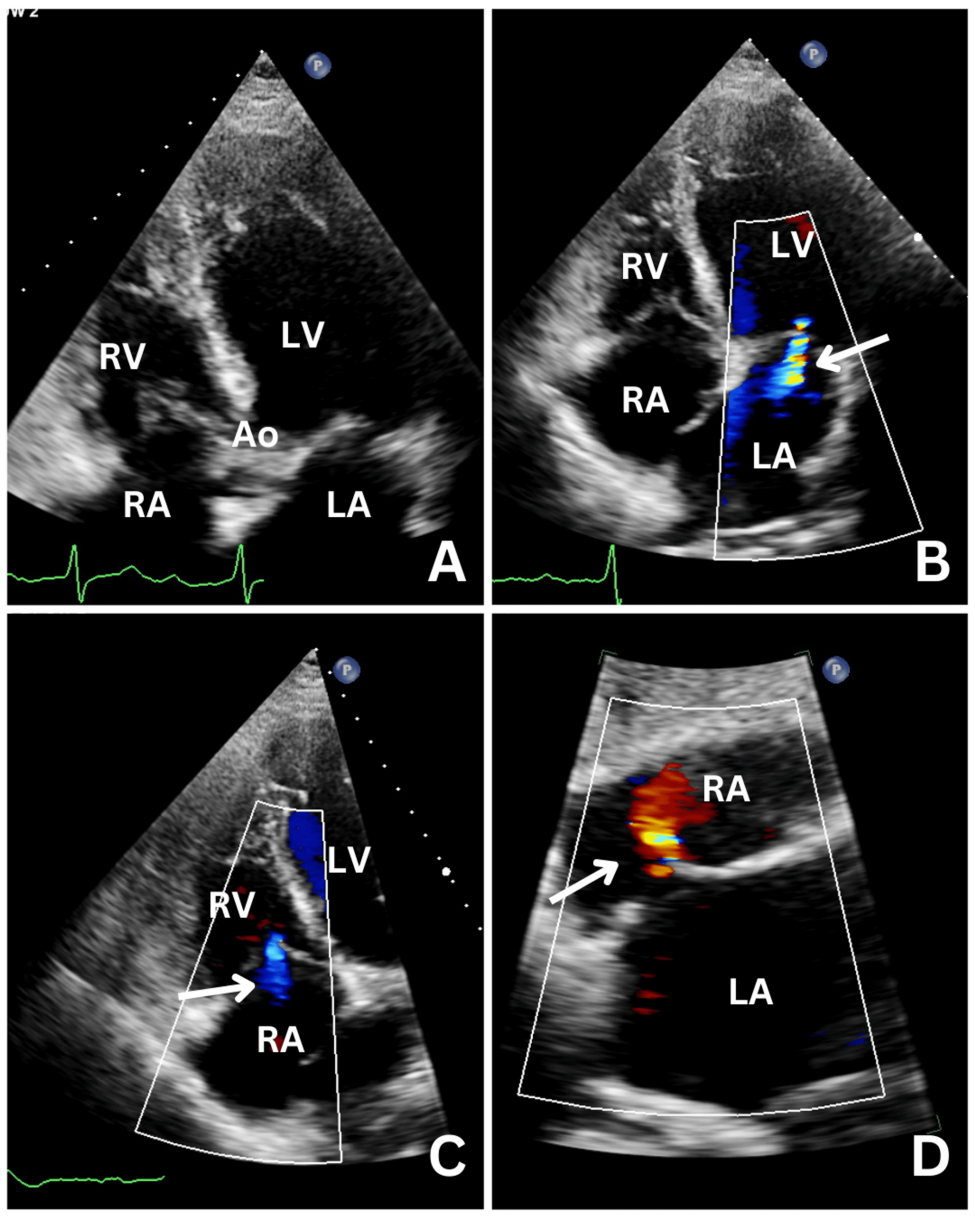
Transthoracic Echocardiography A. Apical four-chamber view shows a dilated Right Ventricle (RV), B. Arrow shows functional mitral regurgitation and dilated atriums in apical four-chamber view, C. Arrow shows mild tricuspid regurgitation and dilated right atrium in apical four-chamber view, and D. Arrow shows a small jet of colour crossing the interatrial septum suggesting the presence of patent foramen ovale (PFO) in subcostal view. LV: left ventricle; LA: left atrium; RA: right atrium; Ao: aorta
